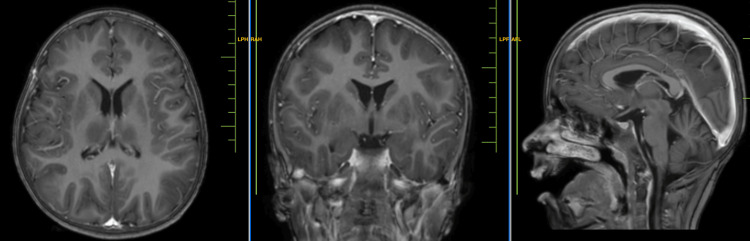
Contrast-enhanced magnetic resonance imaging (CE-MRI) showing no abnormal enhancement.
